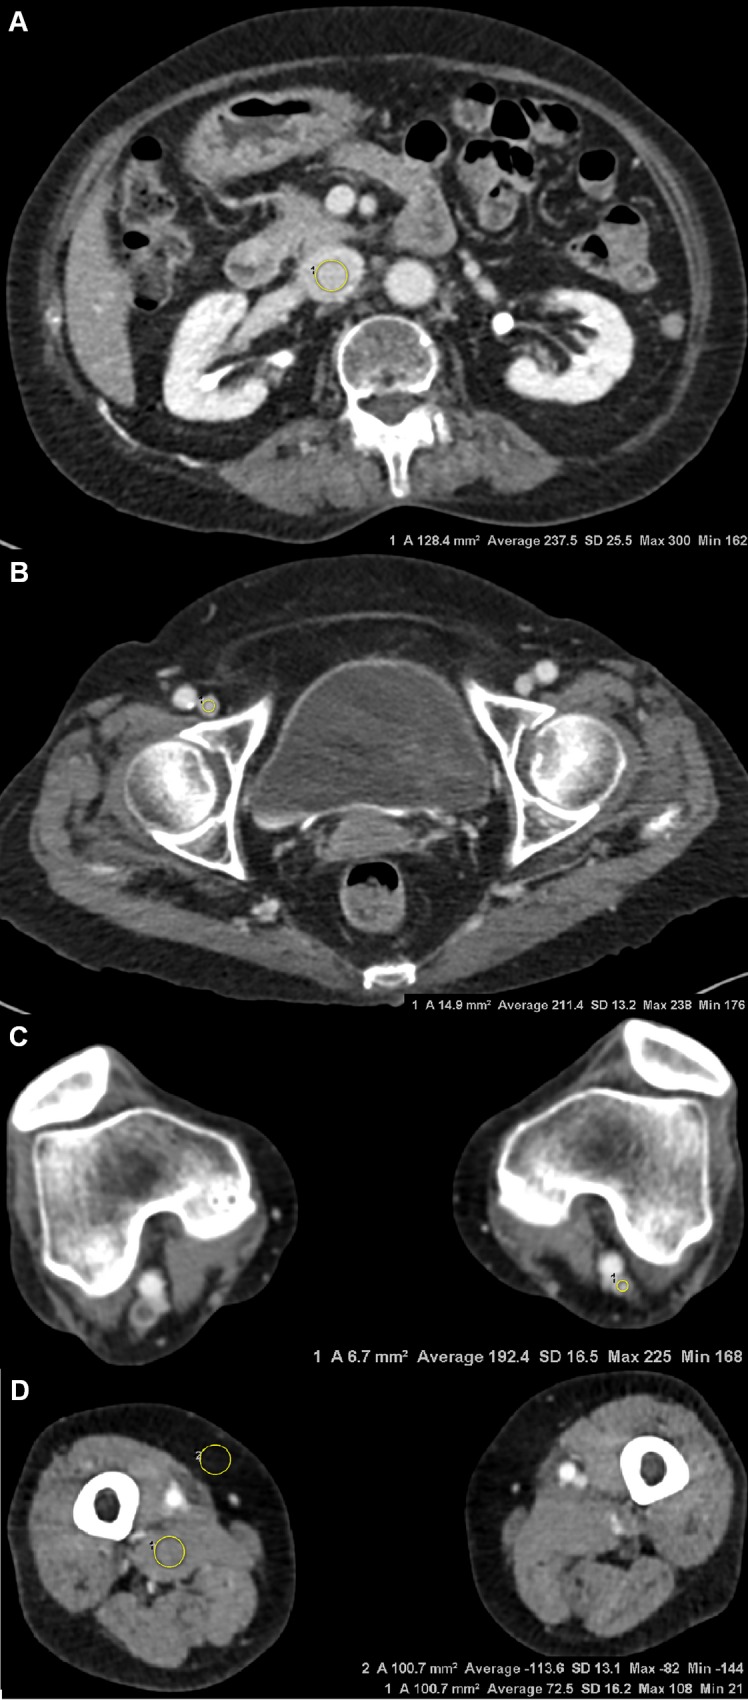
Axial CTV images obtained using 80 kVp and SAFIRE in a 75-year-old woman with BMI 23.2. Attenuation value, vein-to-muscle contrast-to-noise ratio and signal-to-noise ratio in this patient were 237.5 HU, 12.6, and 9.3 in the IVC (a), 211.4 HU, 10.6, and 16.0 in the CFV (b), and 192.4 HU, 9.2 and 11.7 in the PV (c). Attenuation values of subcutaneous fat and muscle at the mid-thigh level were also measured using circular ROIs (d). Intraluminal filling defect (arrow) was detected in the right popliteal vein, which was diagnosed as DVT. The overall image quality scores and image noise scores were 5 (excellent) and 1 (optimal) for reader 1 and 4 (good) and 1 (optimal) for reader 2. *CTV computed tomographic venography, SAFIRE sinogram-affirmed iterative reconstruction, BMI body mass index, HU Hounsfield unit, IVC inferior vena cava, CFV common femoral vein, PV popliteal vein, ROI region of interest.
